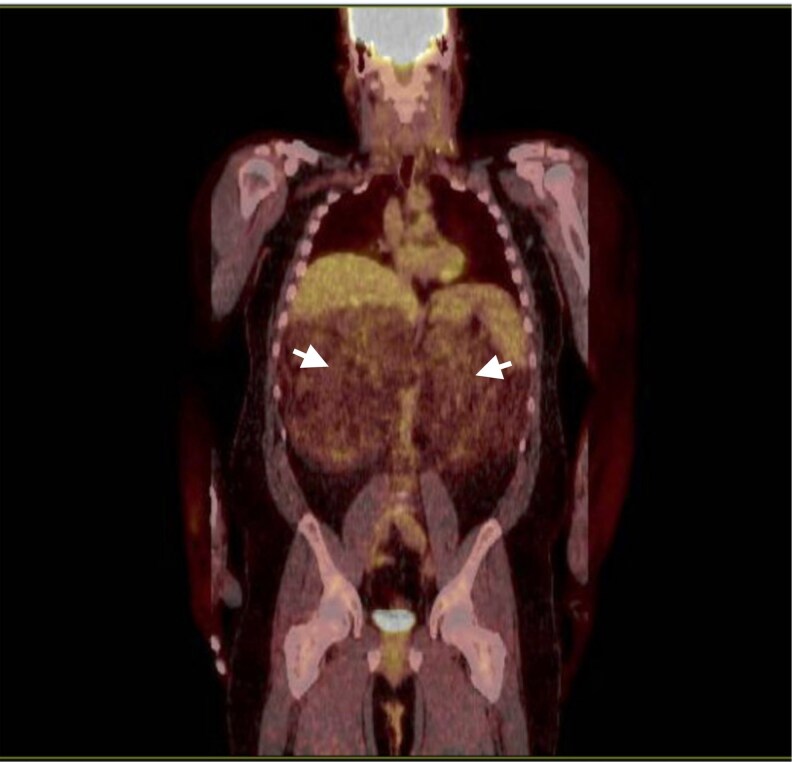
Fluorodeoxyglucose (FDG)-positron emission tomography (PET) scan coronal view. White arrows indicate adrenal masses. This scan was performed before the initial clinic visit and shows bilateral upper retroperitoneal heterogenous masses. Maximum standardized uptake value (SUV) within this retroperitoneal mass measured approximately 2.3, similar to slightly greater than background mediastinum.
